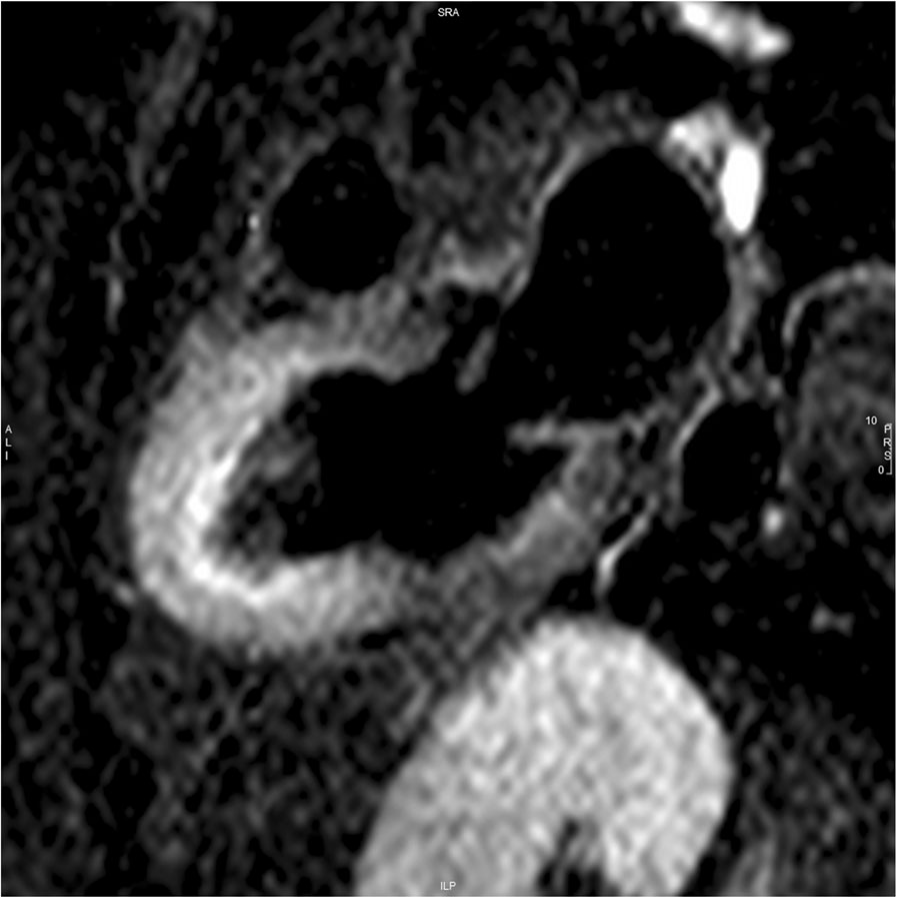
Myocardial edema. T2-weighted triple-inversion recovery 2-chamber view showing transmural signal hyperintensity in the mid-apical segments of left ventricle
